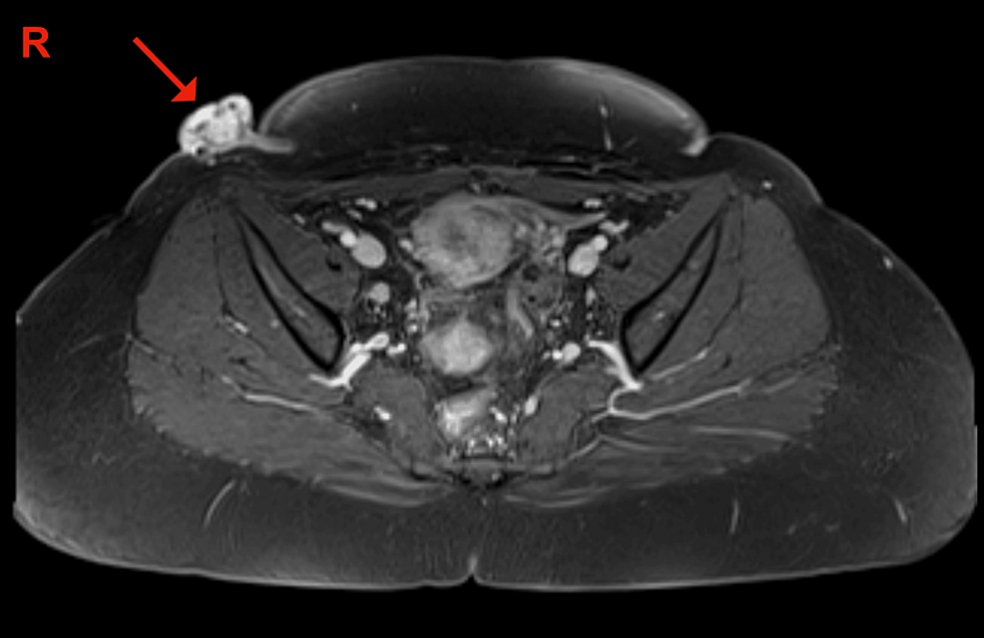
Magnetic resonance imaging (MRI) of the abdomen and pelvis revealing the mass (arrow). It is seen as an intensely enhancing soft tissue mass.
